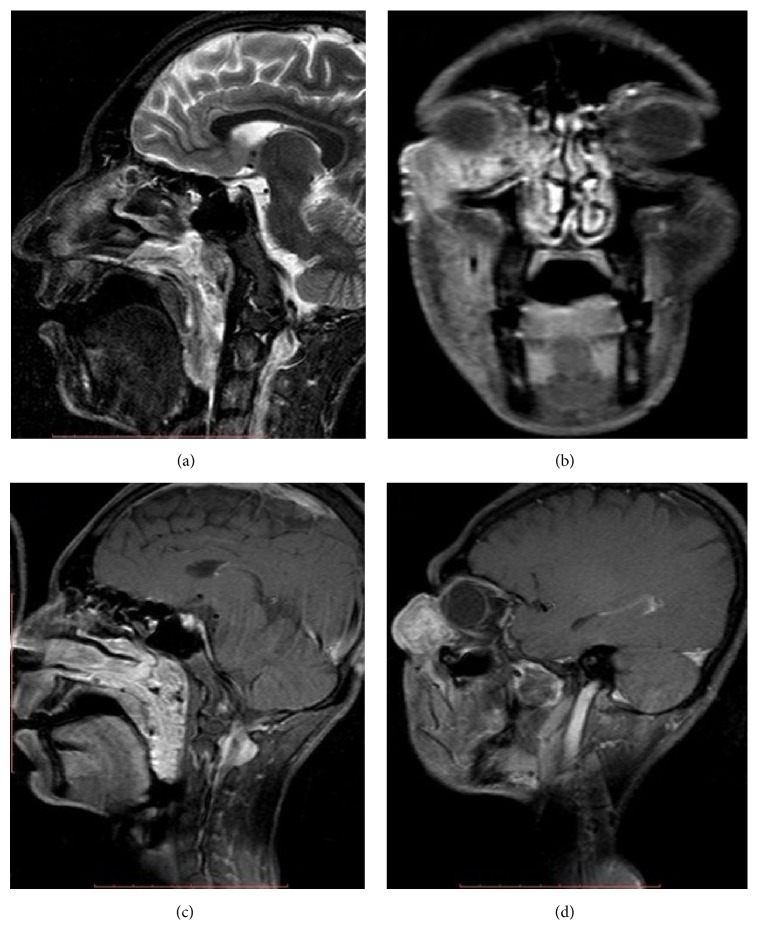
(a) T2-weighted MRI sagittal section showing heterogeneously hyperintense multilobulated mass originating from nasal cavity and nasopharynx and extending into oropharynx. Lesion shows few flow voids within. (b) Postcontrast T1-weighted MRI coronal section showing enhancing mass lesion in inferior portion of right orbit. There are few nonenhancing areas within. Lesion is extending into the inferior turbinate via nasolacrimal duct. (c) Postcontrast T1-weighted MRI sagittal section showing intensely enhancing multilobulated mass originating from nasal cavity and nasopharynx and extending into oropharynx. Lesion shows few nonenhancing areas within. Lesion shows multilobulated external surface mimicking cerebriform appearance of inverted papilloma. (d) Postcontrast T1-weighted MRI sagittal section showing extraconal, intraorbital, intensely enhancing mass extending into the nasal cavity via nasolacrimal duct. The mass is protruding outside of the orbital margins causing proptosis.
